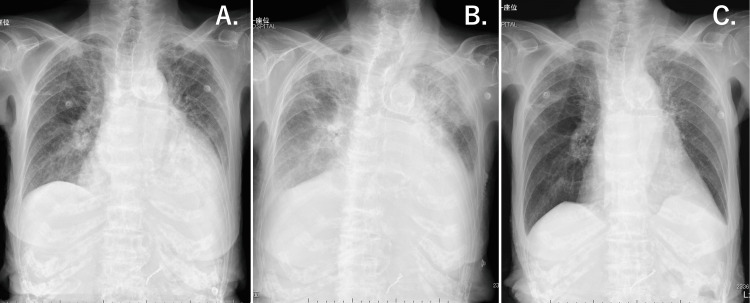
Chest X-ray of the patient (A: day 1, B: day 11, C: day 21)
